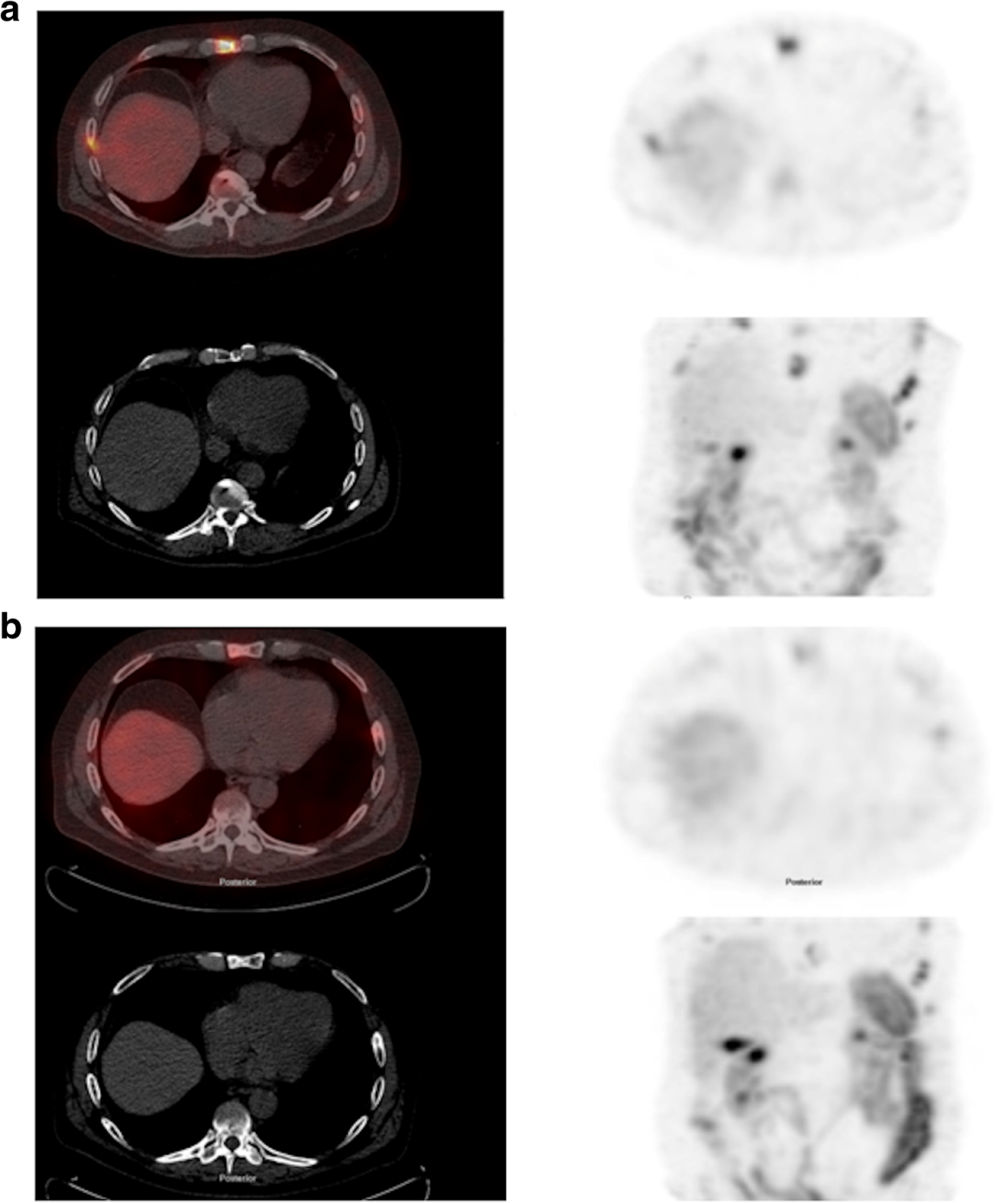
Fused axial SPECT/CT image (*top left*), CT image (*bottom left*), axial SPECT image (*top right*) and coronal maximum intensity image (*bottom left*) show a sternal lesion in a patient with a treatment response: a before treatment (T:N ratio 8.66, T:M ratio 11.97, HU = 155), b 12 weeks after starting treatment (T:N ratio 4.42, T:M ratio 5.18, HU = 413)
